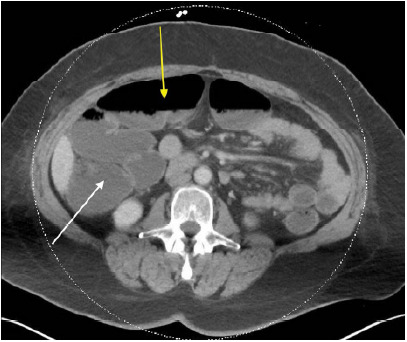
Computed tomography of the abdomen and pelvis (axial) demonstrating dilated large bowel/ascending colon (white arrow) with air-fluid levels (yellow arrow).
